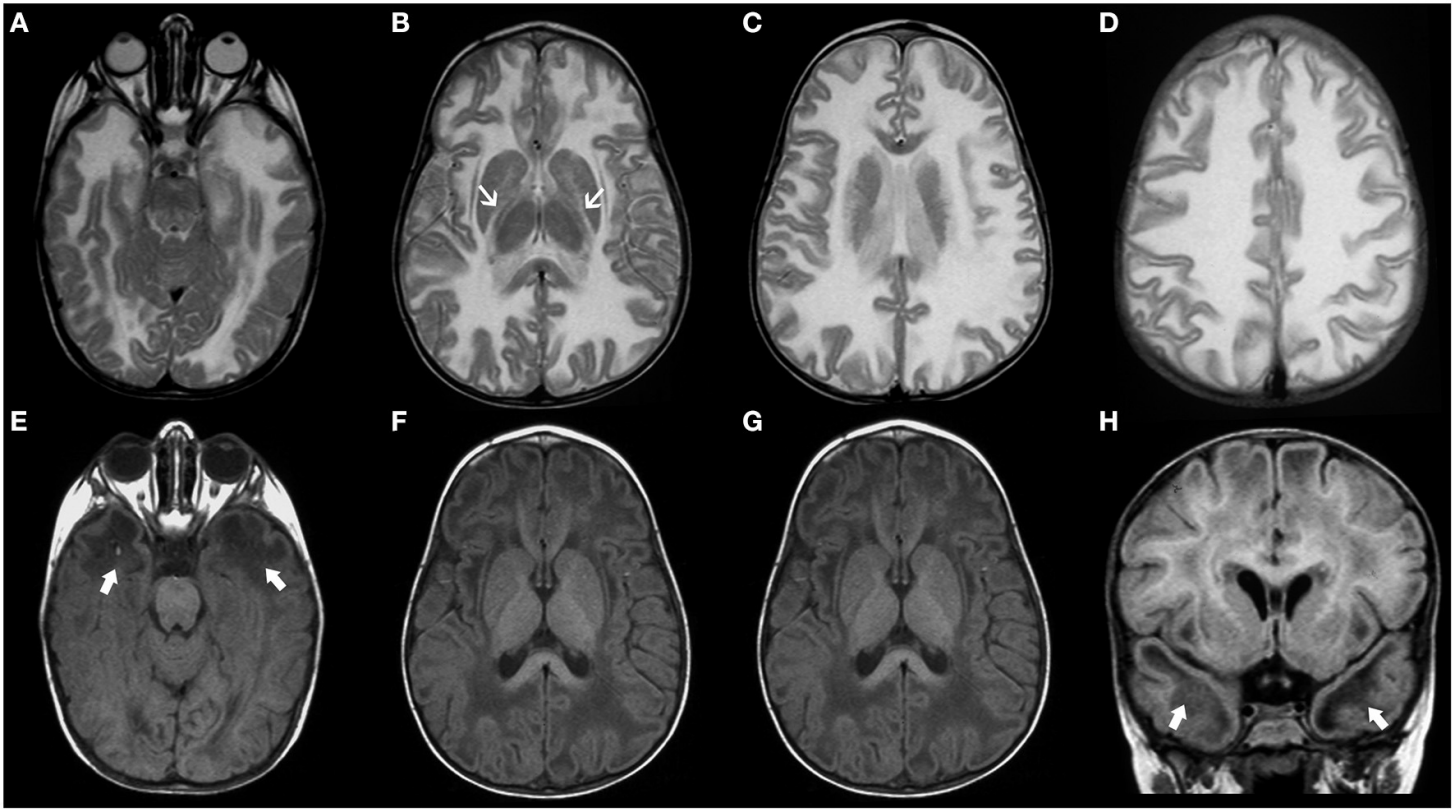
Imaging characteristics of Megalencephalic Leukoencephalopathy With Subcortical Cysts (classic form). Axial T2WI (A–D), axial (E,F), and coronal (G) T1WI, and coronal FLAIR (H) performed in an affected boy at 1.8 years of age show diffuse, bilateral, and symmetric cerebral white matter hyperintensity including the subcortical white-matter/U-fibers, leading to brain swelling and effacement of the cortical sulci. There is a characteristic double-line signal abnormality in the posterior limb of the internal capsule (white arrows), i.e., a residual central dark line surrounded by two strands of hyperintensity in this location. Also note the typical areas of cystic-like white-matter rarefaction in the temporal-polar regions on T1WI and FLAIR (thick white arrows).
